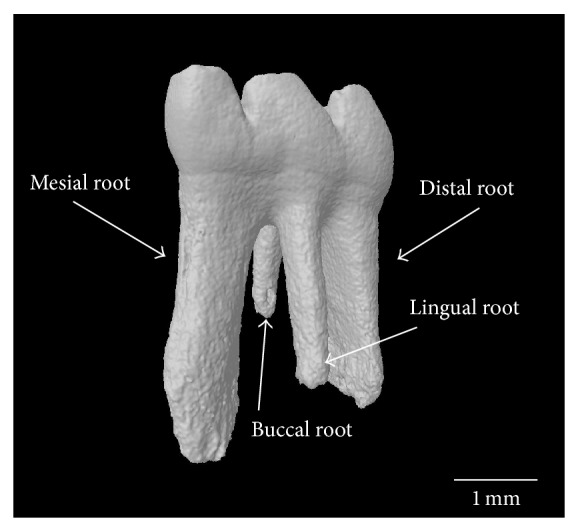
μ*-CT 3D reconstructed image of the four roots of molar 1 (M1). The mesial, distal, buccal, and lingual roots enclose the alveolar bone of the interradicular septum (image by B. Johnston).
